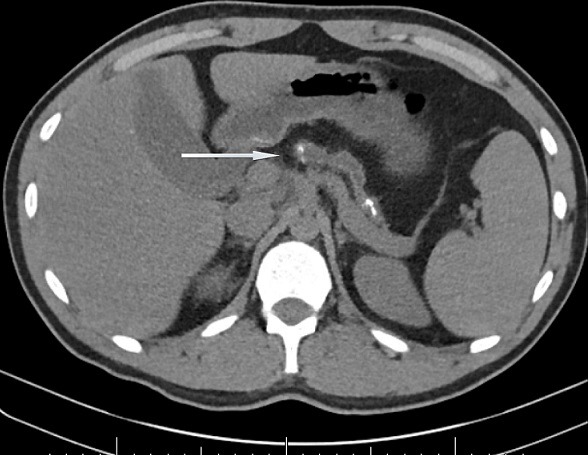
CT abdomen showing pancreatic atrophy with multiple sub-centimeter calcifications consistent with severe chronic pancreatitis: arrow points to dilated pancreatic duct with stones in it.
